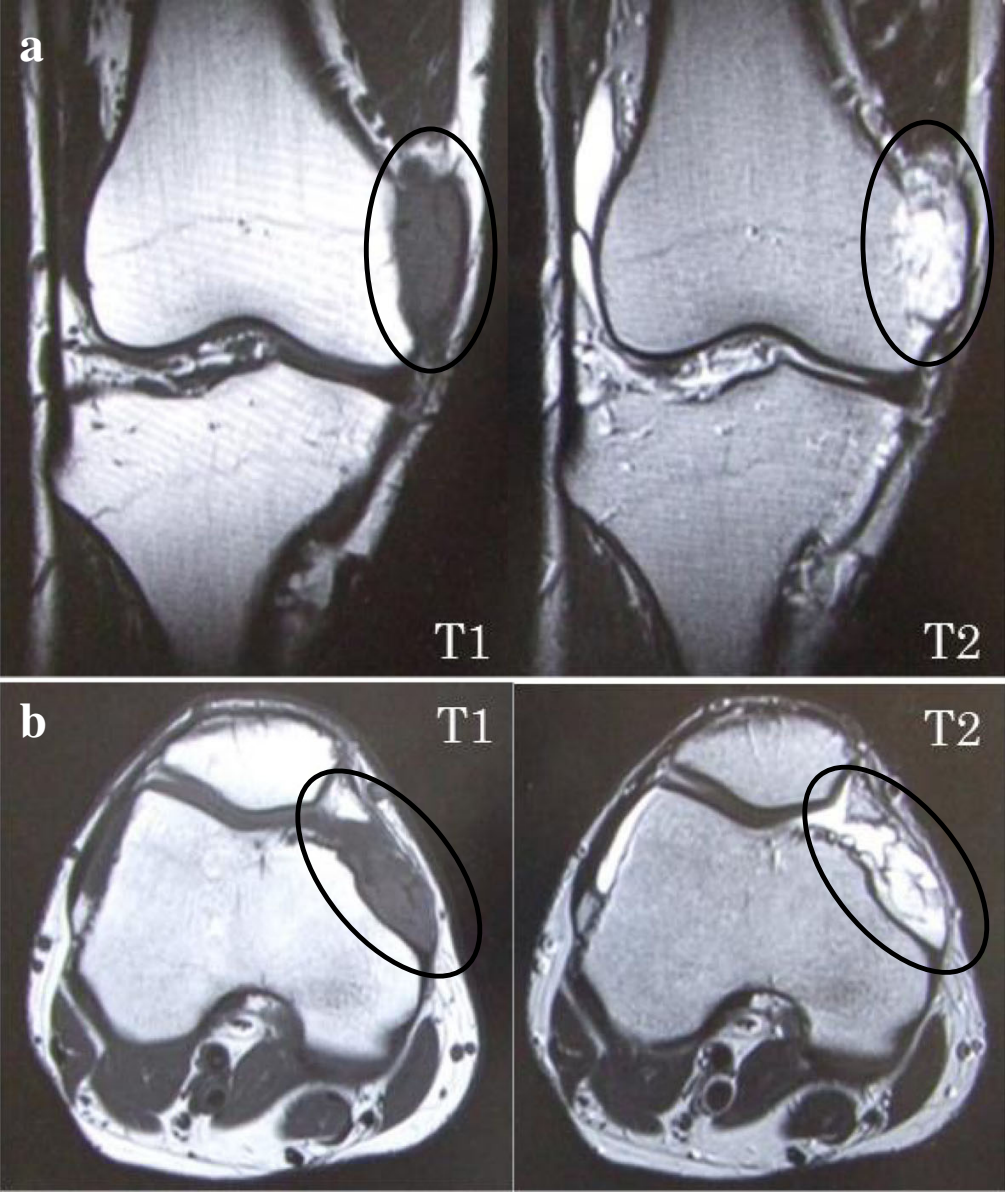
Magnetic resonance (MR) (a) coronal image and (b) axial image. MR images show a mass (circle). On axial T2-weighted MR images, the coronal view indicates an intra-articular mass from the patellofemoral to medial femorotibial joint level. Axial T1 image shows that the mass is homogeneous with low intensity. Axial T2 image shows that the mass is homogeneous with a high-intensity lesion. The high-intensity lesion is adjacent to the medial femoral condyle. The margin of bone is irregular. The ovals indicate the mass
